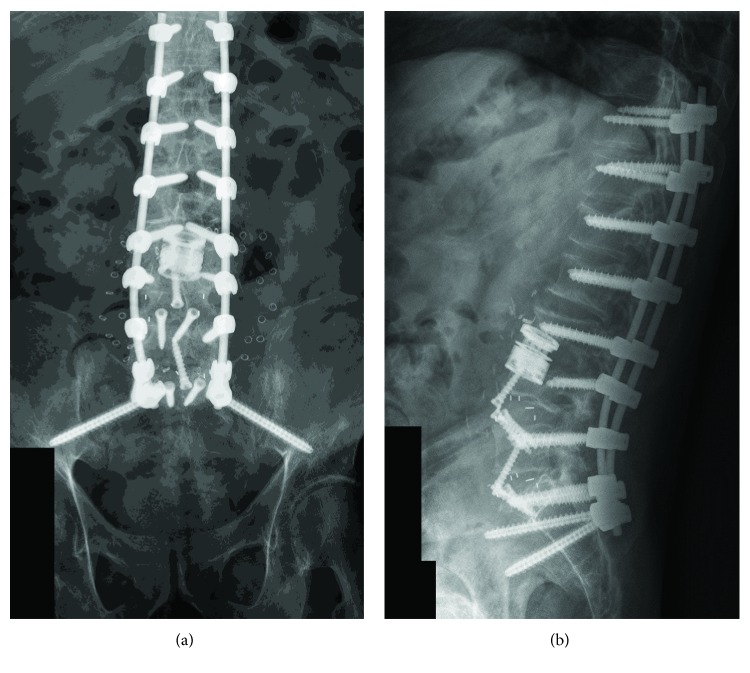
(a, b) Standing anterior-posterior and lateral X-rays demonstrating T11-S2 bilateral pedicle screw and rod fixation with bilateral iliac screws. Anterior interbody L4 and L5 PEEK graft. Anterior L3 intradiscal expandable cage and bone graft.
